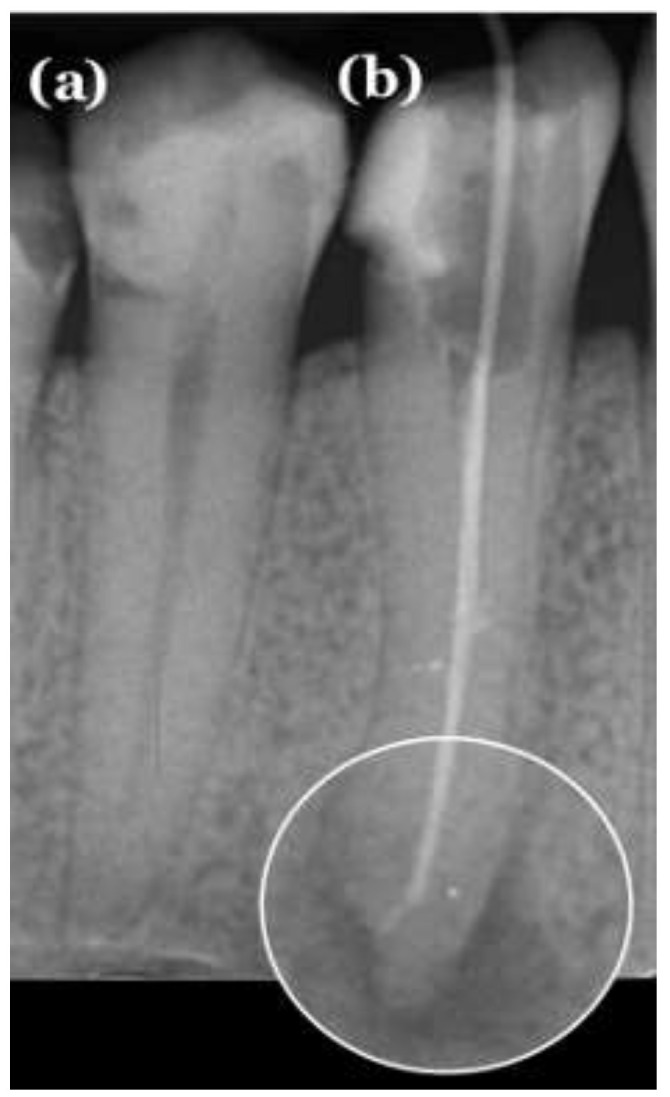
Periapical radiograph showing two mandibular premolars with the periodontium. The investigators classified the teeth as (a) healthy and (b) unhealthy.
